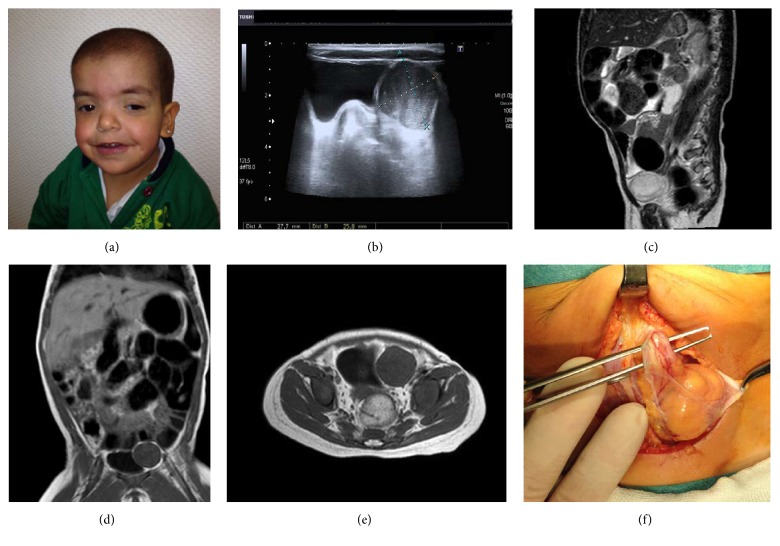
Patient 1. (a) Photograph of the physical features of the patient with low-set ears, lower palpebral fissures, hypertelorism, broad nasal bridge, thick lips, and thin hair. (b) Abdominal ultrasound showing the presence of a solid mass paravesical displacing the left ovary and bladder without infiltrate surrounding structures. (c) Initial MRI showing a hyperintense tumor predominantly heterogeneous intensity on T2-weighted sequence compressing the bladder. ((d) and (e)) MRI on T1-weighted sequences after 3 cycles of chemotherapy with slight decrease in left paravesical oval mass. (f) Operative image indicating the presence of tumor originating in the left medial UL.
